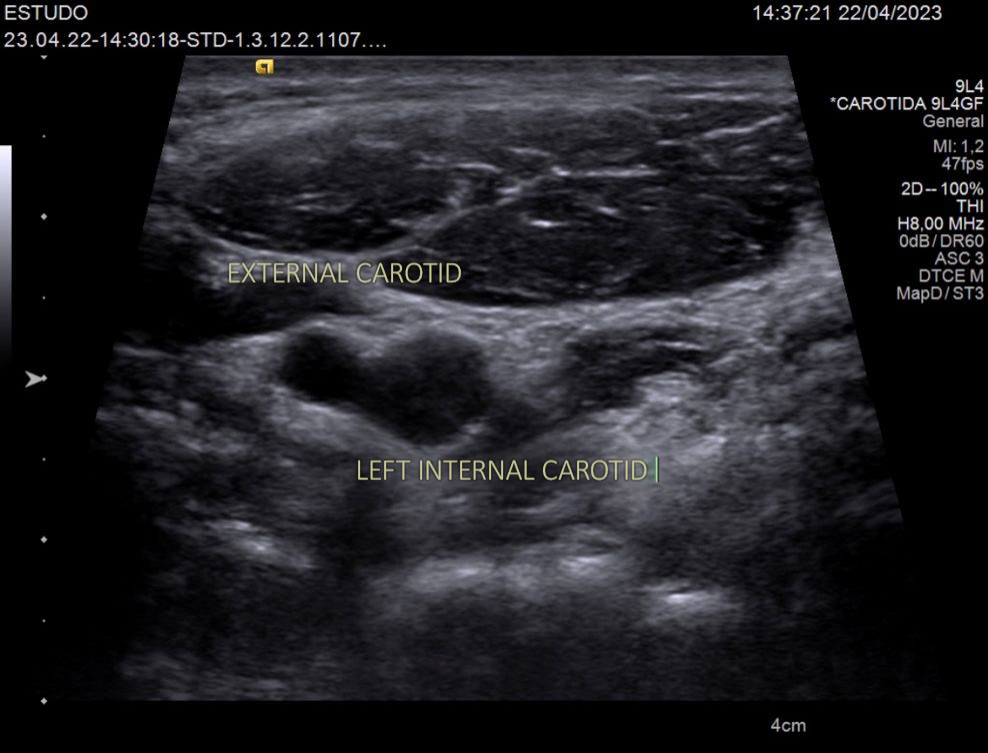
Transverse ultrasound image showing the left carotid bifurcation. The left external carotid artery and left internal carotid artery are being analyzed.
